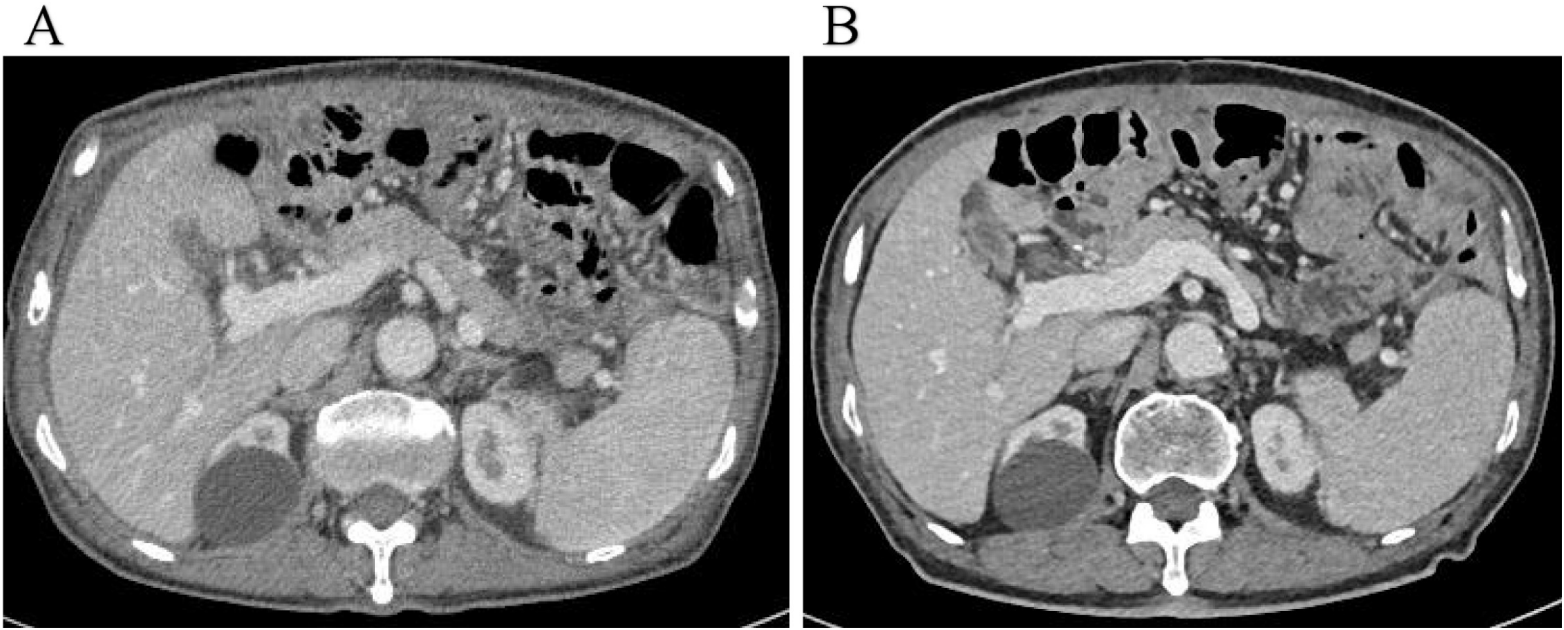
(A, B) Surveillance abdominal CT scans performed in May and October 2024 showed no recurrence of ascites.
